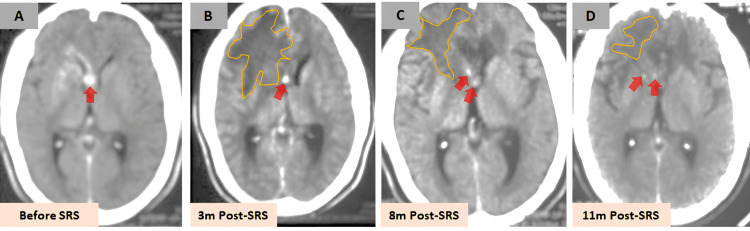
Serial CT follow-up post-SRS Serial CT images before and after SRS demonstrating a progressive treatment response. (A) Baseline CT before SRS. (B) At three months post-SRS, there is a slight reduction in arteriovenous malformation nidus size (red arrow), with significant vasogenic edema in the right frontal region (yellow outline). (C, D) Both the edema and nidus gradually decreased over time. SRS, stereotactic radiosurgery
